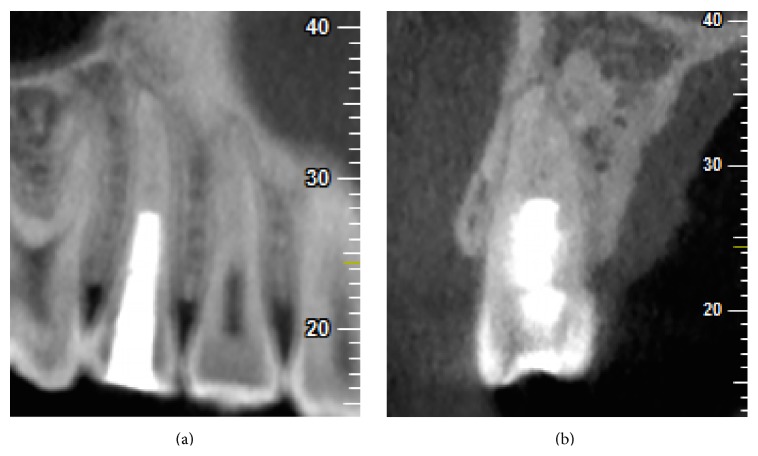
CBCT images at 66 months showing the presence of a narrowed canal lumen due to severe calcifications. (a) CBCT (sagittal view). (b) CBCT (coronal view).
